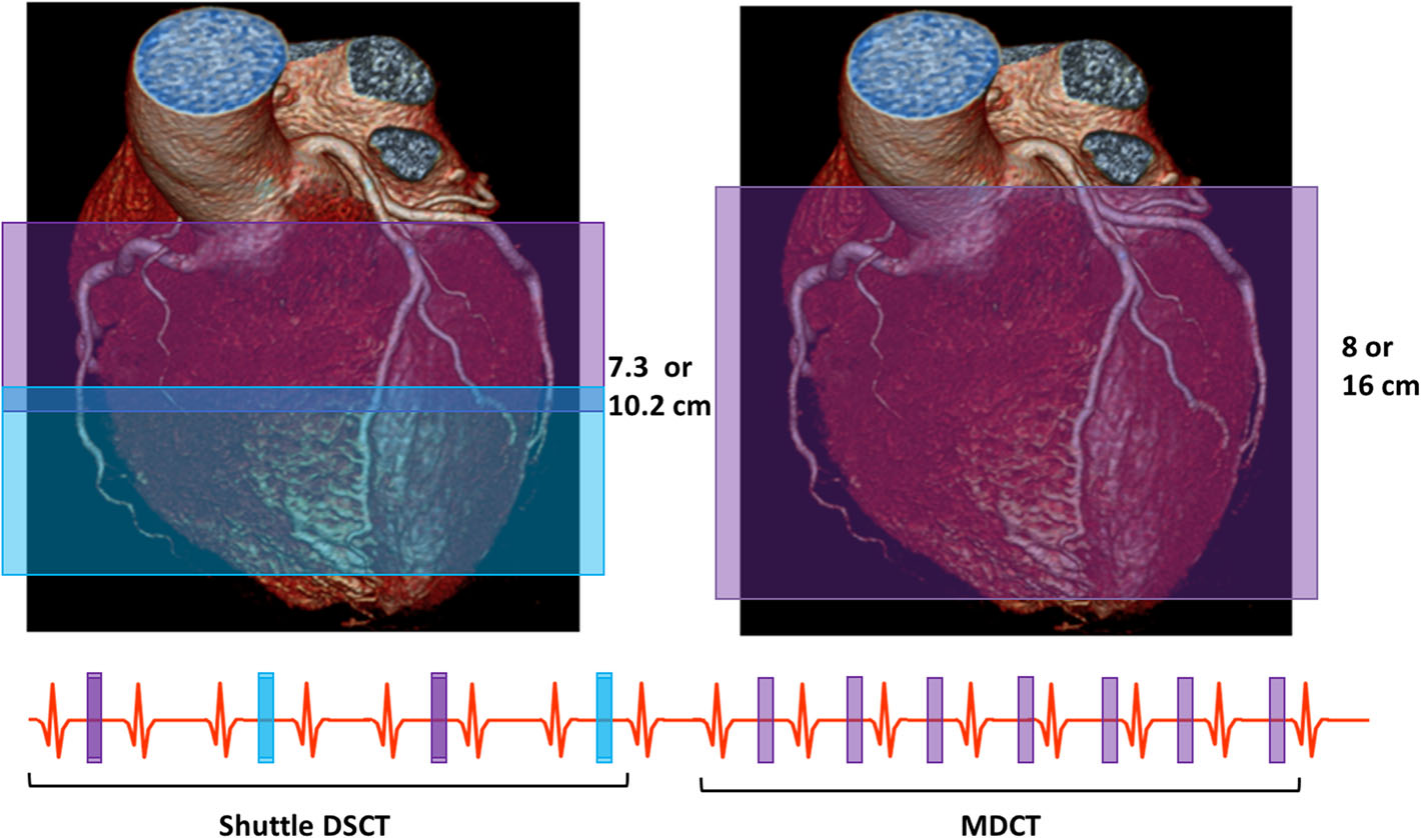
Dual-source CT uses a shuttle mode, alternating table position using two acquisitions merged into one image, to get a *z*-axis of 7.3 or 10.2 cm for the second- and third-generation systems. Multi-detector CT systems use wide detector with 280 or 320 rows to reach a *z*-axis range of 8 or 16 cm, respectively. Both approaches allow for whole-heart imaging
